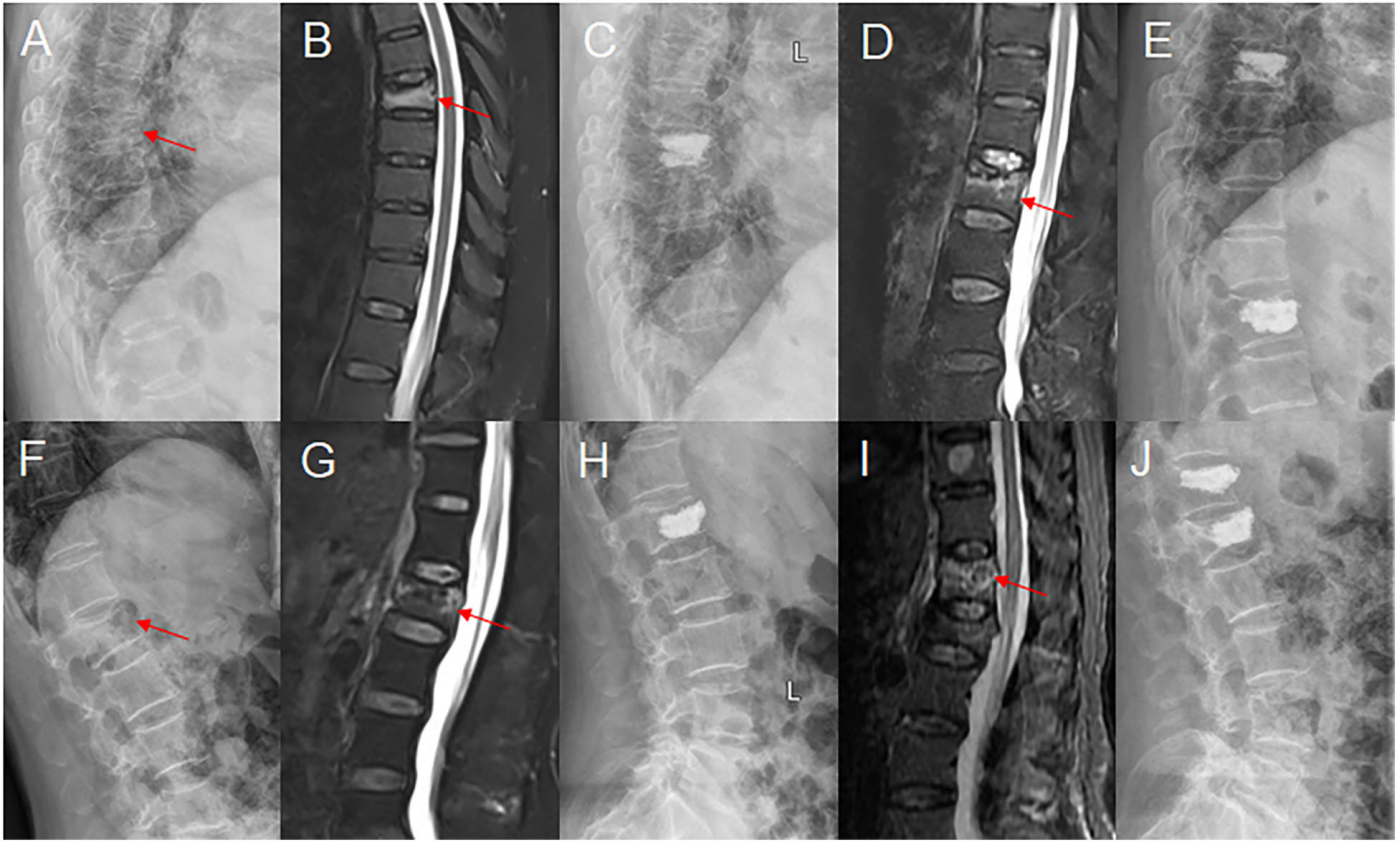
Two typical cases of SVF after PVP (A-E) and PKP (F-J), respectively. (A, B) T7 fractured vertebrae was showed on X-ray and MRI. (C) T7 vertebrae treated with PVP was observed on X-ray. (D) MRI revealed T12 vertebrae fracture as SVF. (E) T12 vertebrae filled with bone cement was revealed on X-ray. (F, G) L1 fractured vertebrae was found on X-ray and MRI. (H) L1 vertebrae treated with PKP was observed on X-ray. (I) MRI revealed T12 vertebrae fracture as SVF. (J) T12 vertebrae filled with bone cement was showed on X-ray.
